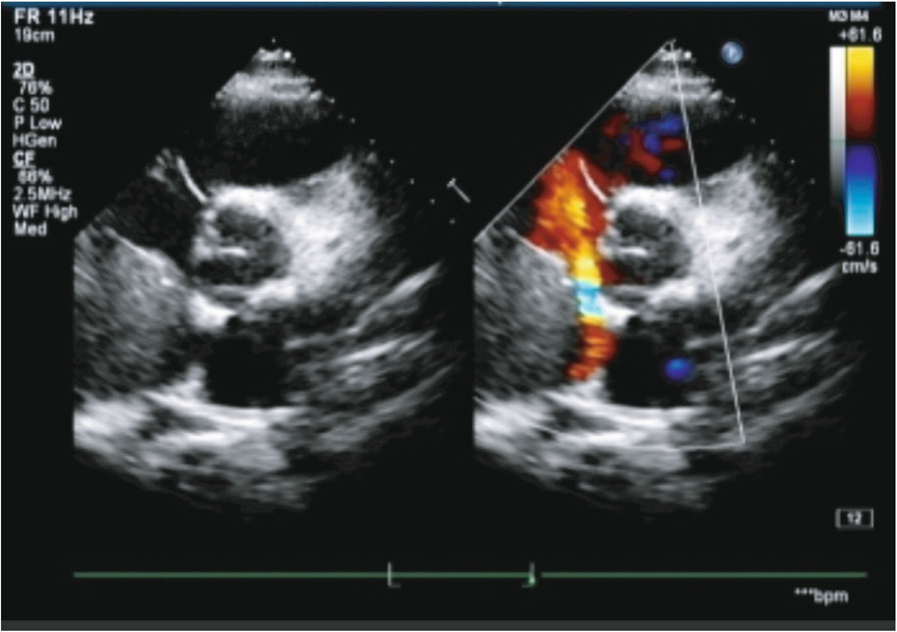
Echocardiography shown existing IVC thrombus had extended into the right atrium
